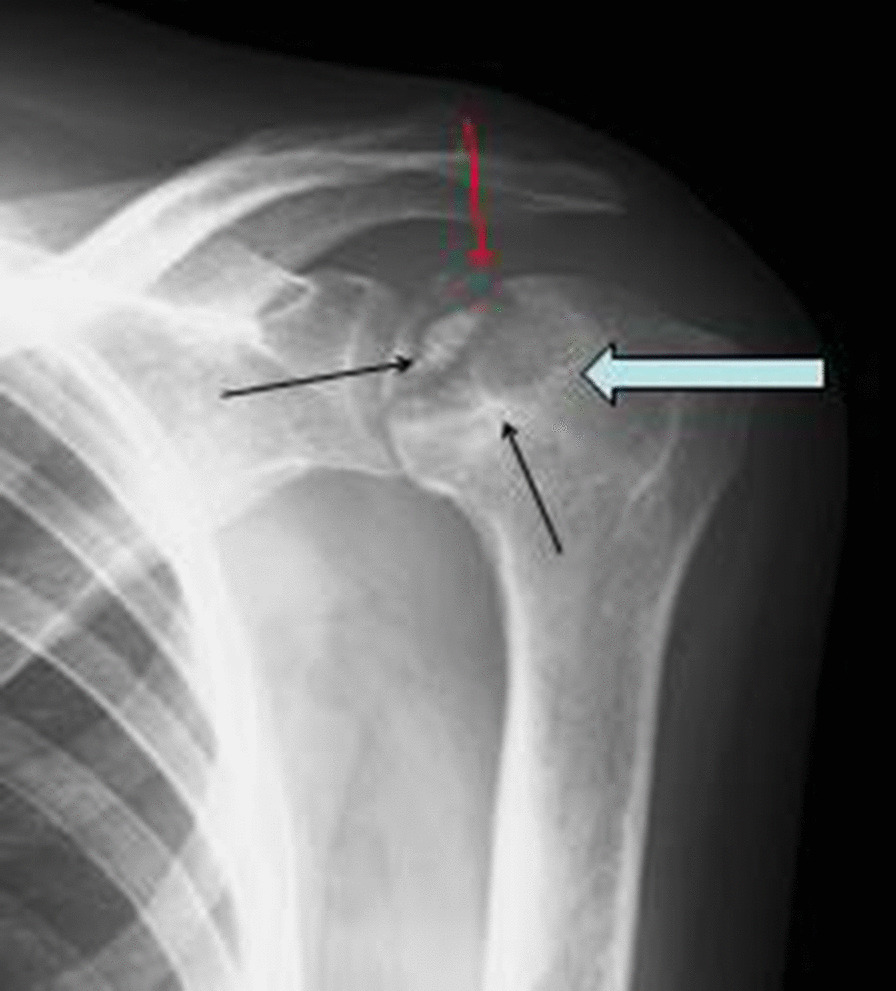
The left shoulder x-ray showed a patch of osteolysis (thick blue arrow) on the humeral head with a clear
